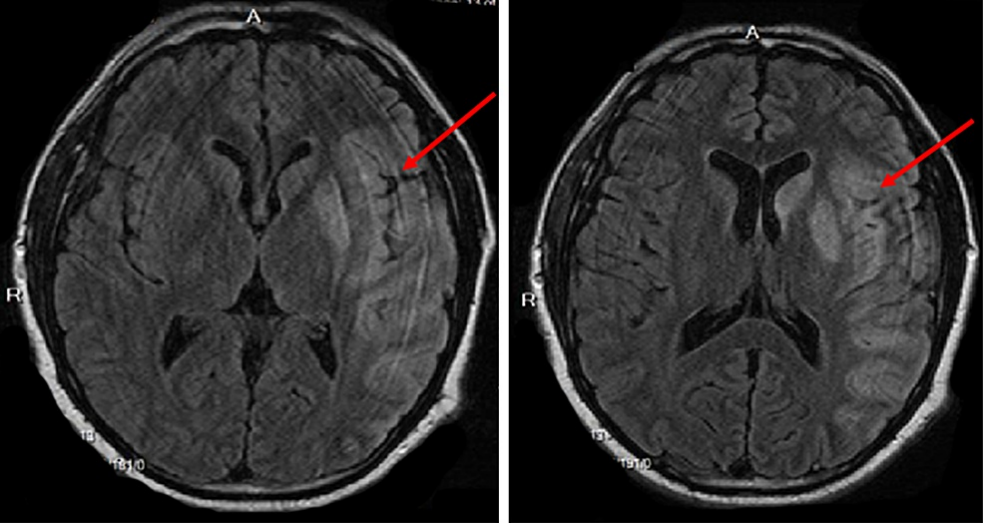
Magnetic resonance imaging (MRI) of the brain showing hyperintense areas in the left frontotemporal and parietal lobes including globus pallidus
